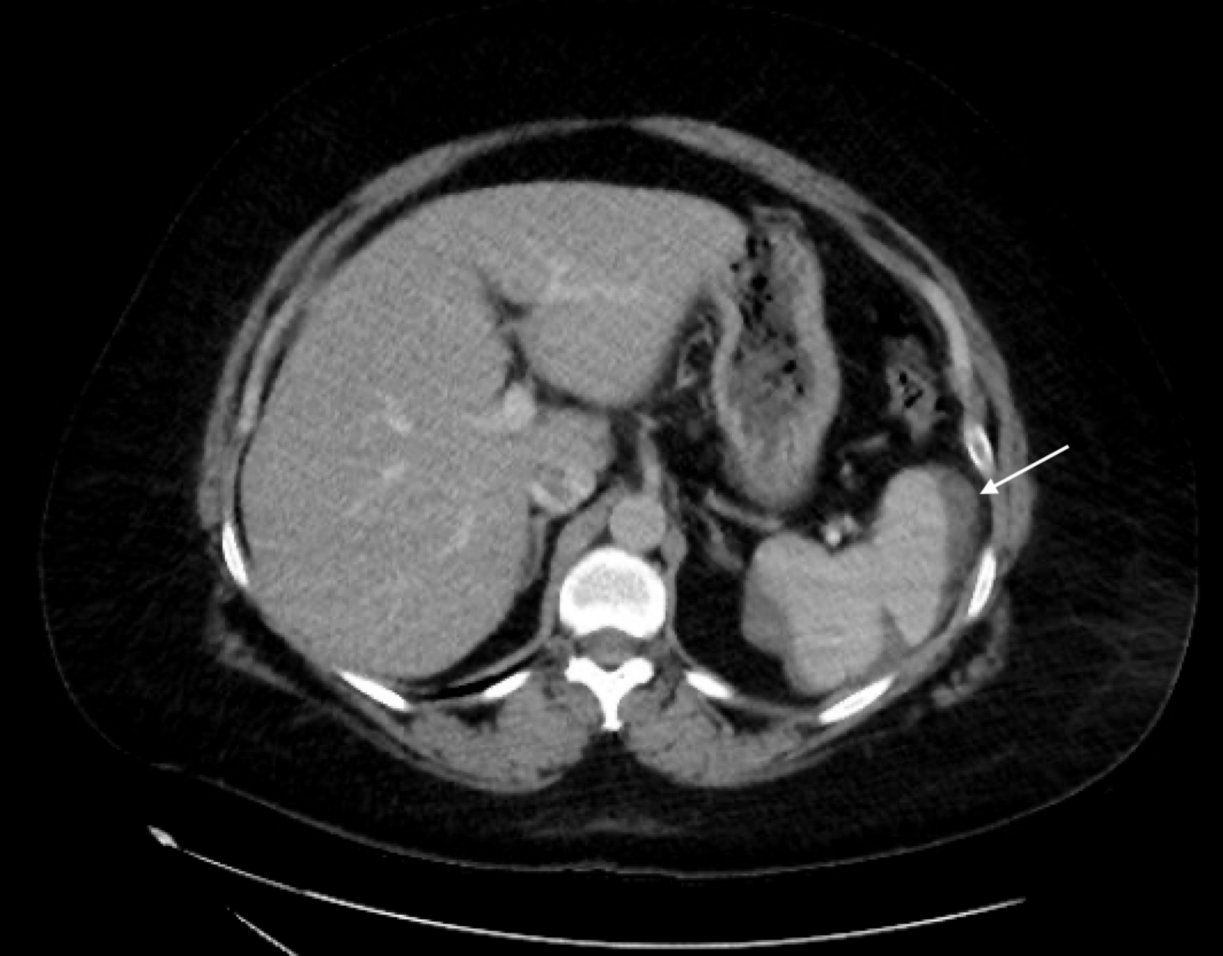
Repeat CT abdomen consistent with a pericapsular hematoma contouring the peripheral spleen appearing smaller and less dense
